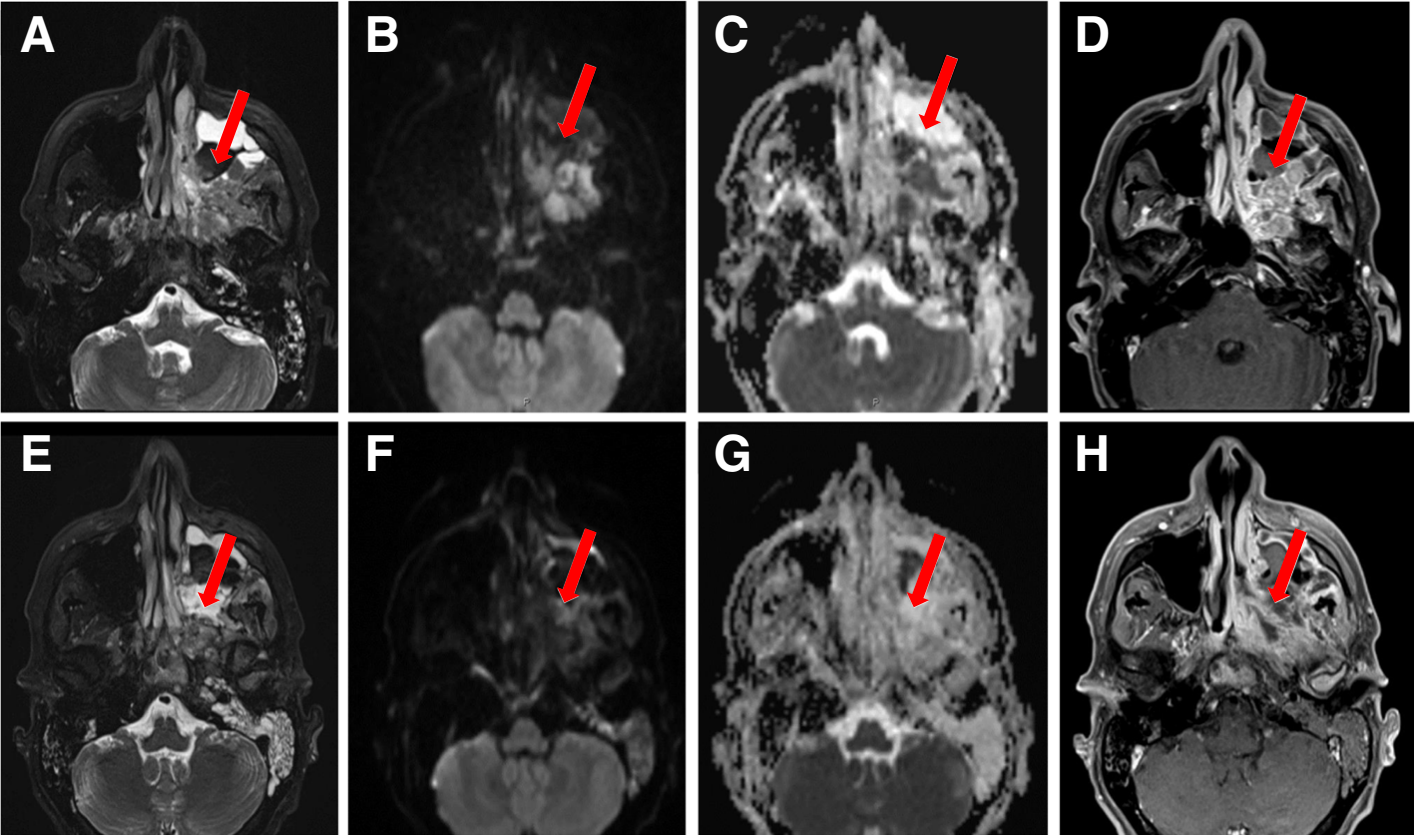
Local tumor response seen in head and neck magnetic resonance images. Magnetic resonance images from baseline before second-line chemotherapy (a–d) showing locally advanced left maxillary sinus mass (*arrows*), infiltrating adjacent sinuses and skull base foramina. Follow-up magnetic resonance images (e–h) showing significant tumor shrinkage (*arrows*) with areas of necrosis, representing local morphological partial response
